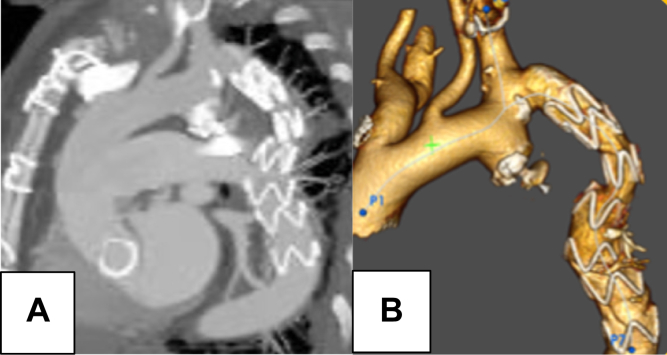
One-year follow-up CT angiography. Follow-up CT scan at 12 months showing stable endograft position, complete thrombosis of the pseudoaneurysm sac, and absence of complications or endoleaks (A). CT angiography reconstruction (B). *CT*, Computed tomography.
